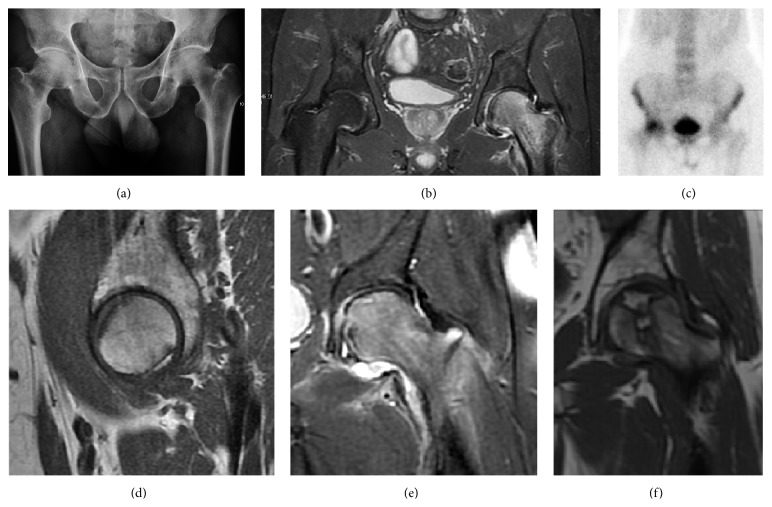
(a) X-ray of a patient with transient osteoporosis of the left hip showing osteoporosis. (b) Coronal stir imaging showing diffuse edema. Scintigraphy (c), sagittal T1 (d), and coronal PD fat sat (e) of a patient with a subchondral fracture of the femoral head with convex shape to the articular surface. Coronal T1 (f) of a patient with avascular necrosis of the femoral head.
